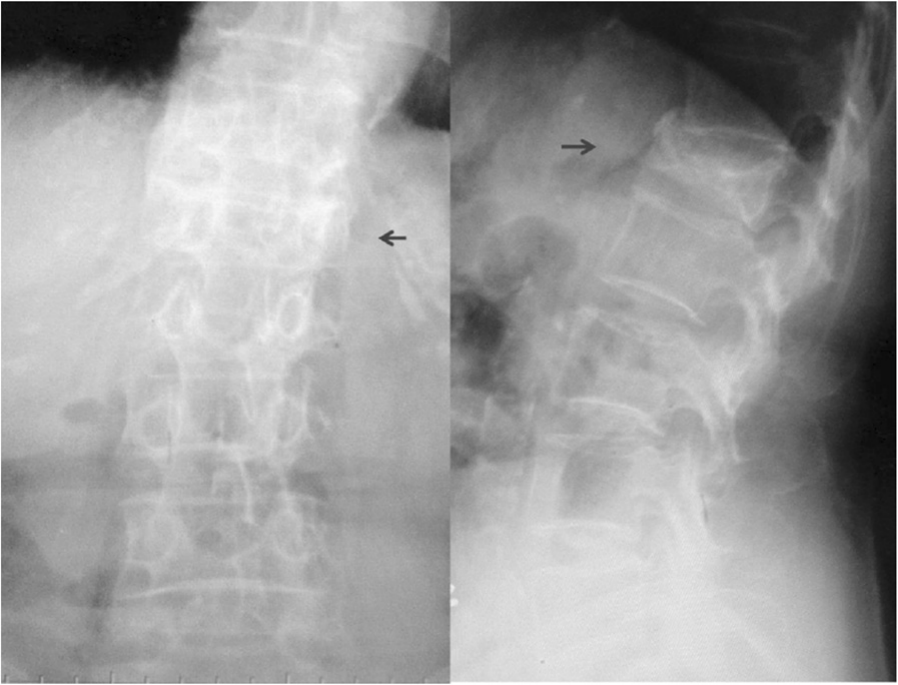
X-ray in a 70-year-old SCN patient. A collapsed vertebral fracture at L1 is shown (arrow).
